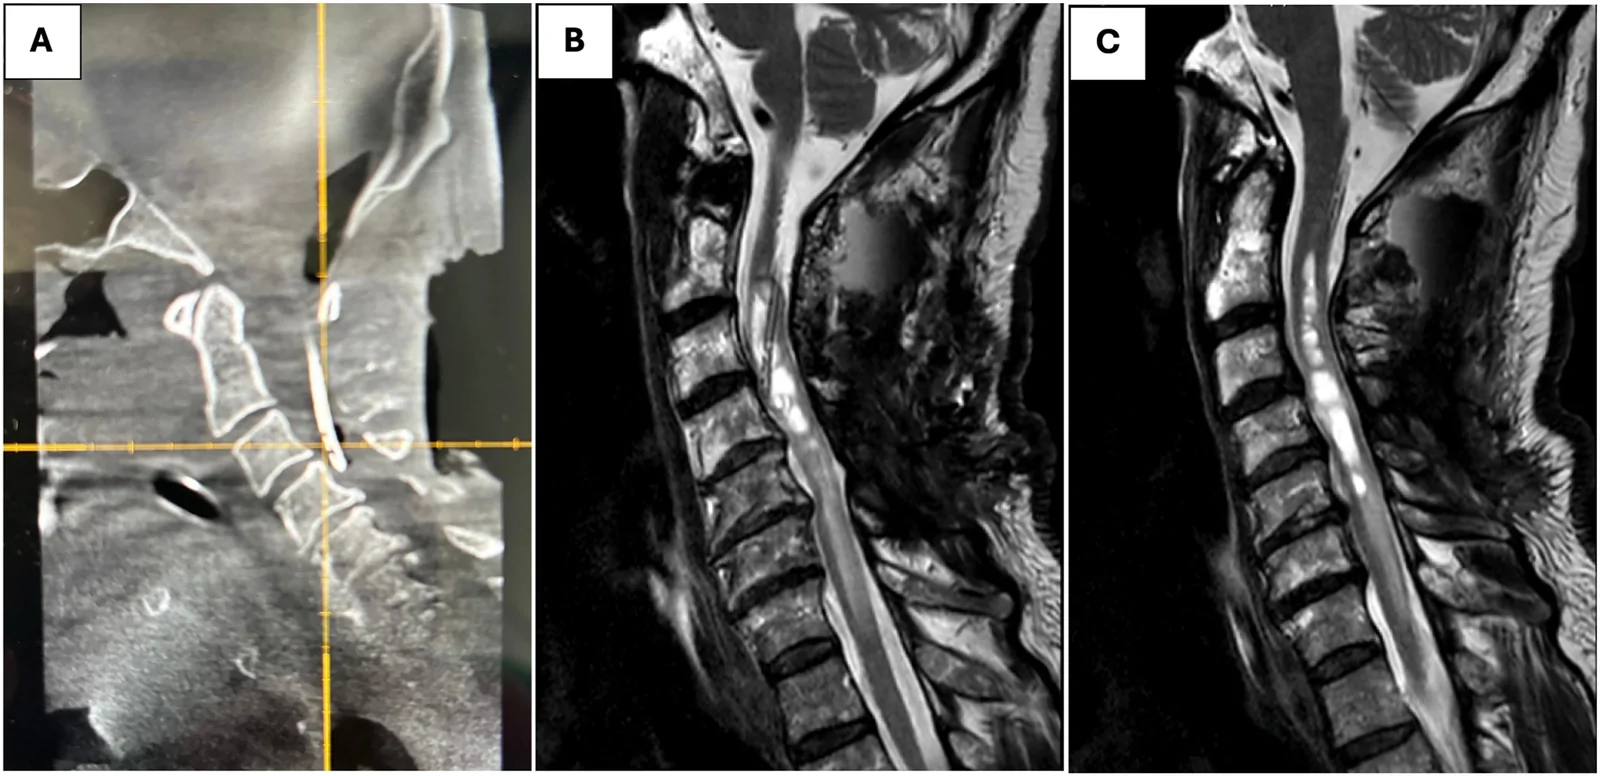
Intraoperative CBCT following VA shunt implantation. (A) Axial scan and (B) coronar reconstruction confirming correct distal catheter tip position within the right atrium following insertion via the internal jugular vein (white arrows), with no evidence of malposition, extravascular course, pneumothorax, or other intrathoracic complications. Intraoperative CBCT of the brain fused with preoperative MRI (C) demonstrating correct positioning of the ventricular catheter in the ipsilateral ventricle.
